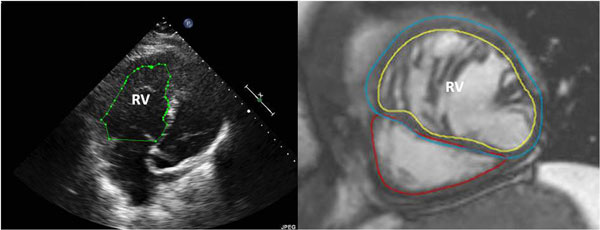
Measurement of right ventricular (RV) end diastolic area by echocardiogram (panel 1) and measurement of RV end diastolic volume by cardiac MRI (panel 2).
